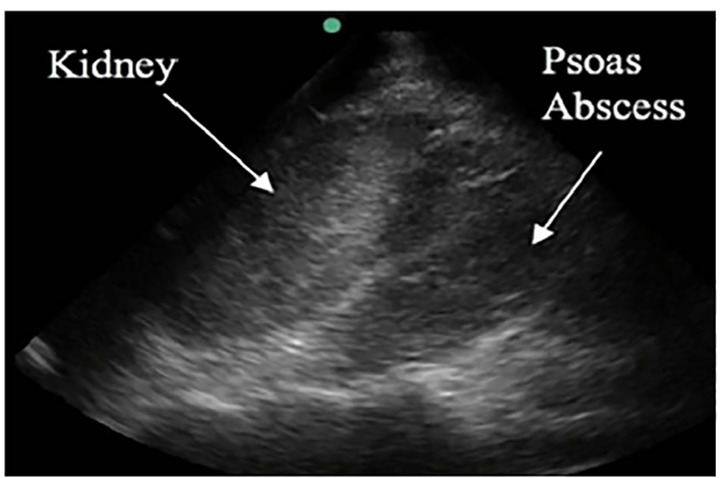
Right upper quadrant ultrasound demonstrating heterogenous mass within the psoas musculature.
